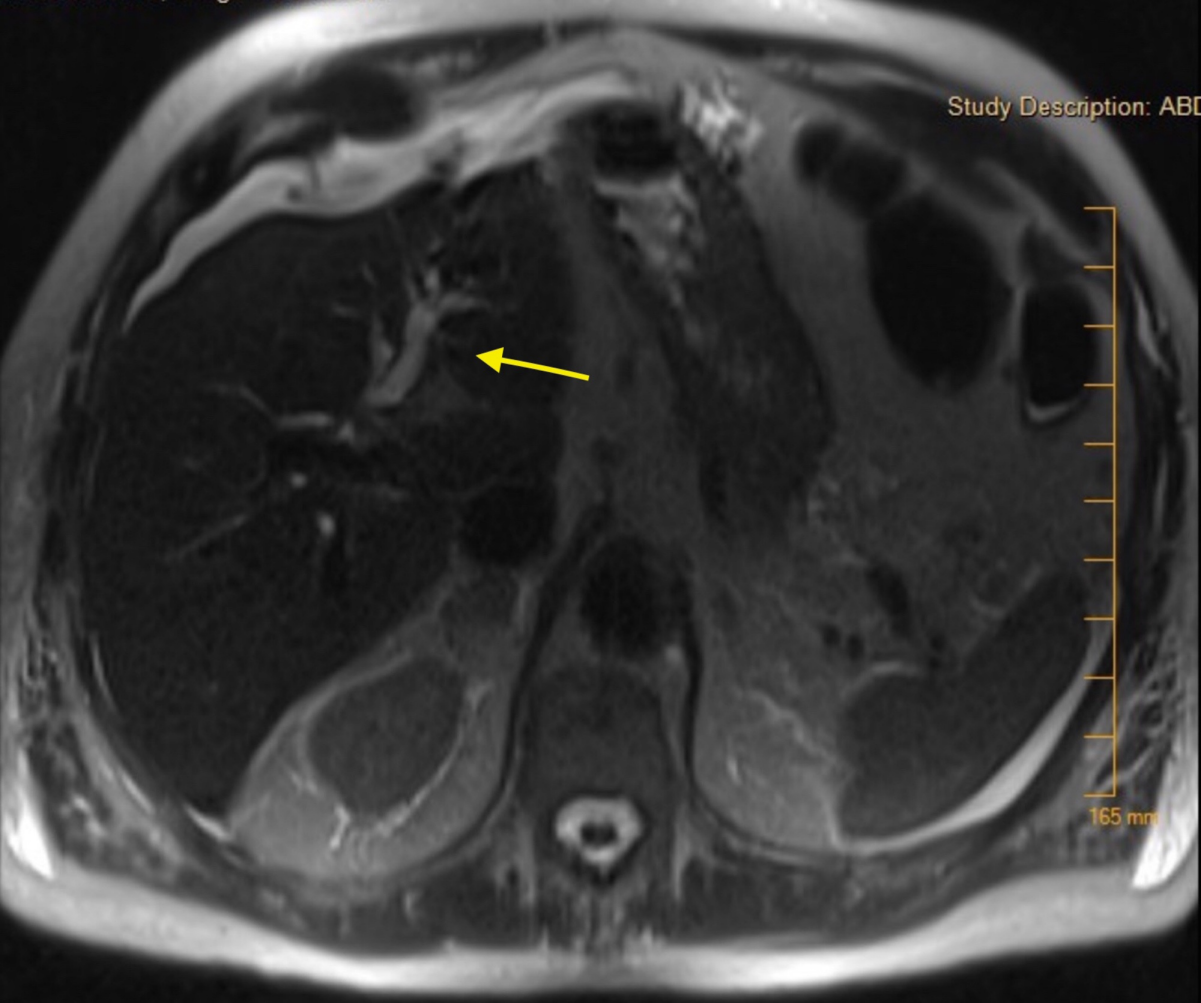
MRI of the abdomen and pelvis demonstrating dilation of biliary ducts (arrow) MRI: magnetic resonance imaging
